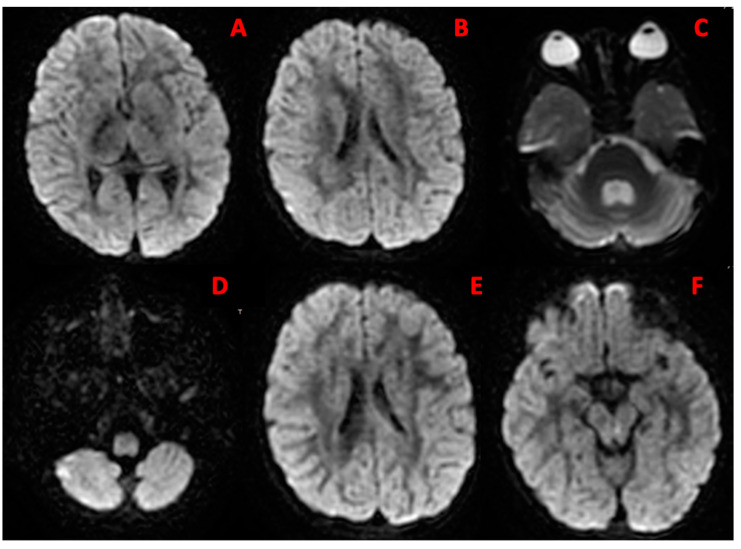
Brain MRI features. Brain MRI features: (A,F) gray matter reduction; (B,E) enlargement of the lateral ventricles; (C,D) cerebellar atrophy.
